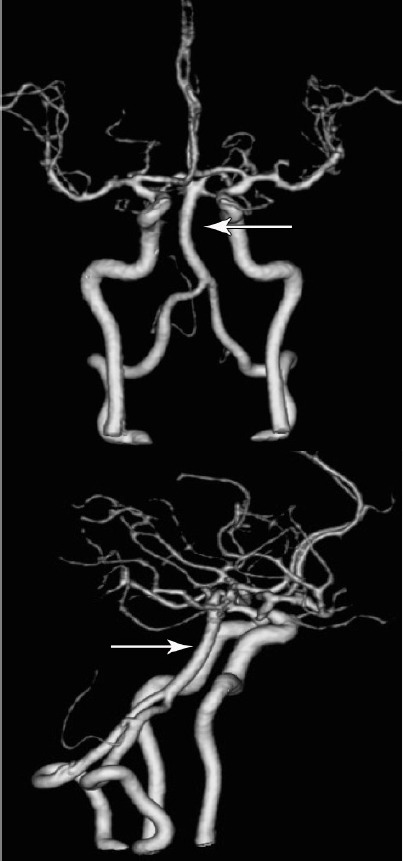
Anterior-posterior and lateral views CTA 3D reconstruction shows complete resolution of basilar artery vasospasm in response to milrinone therapy.
